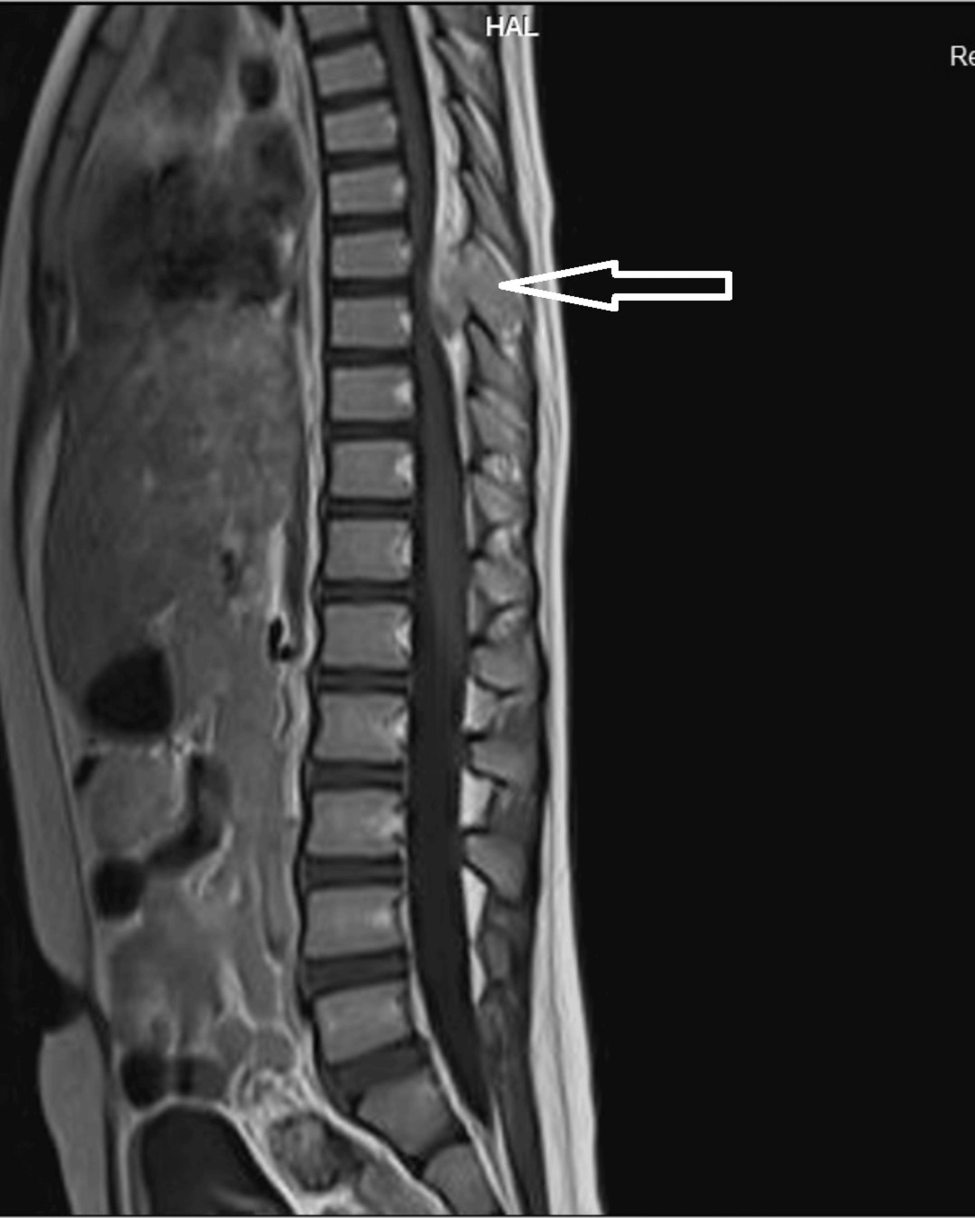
MRI image showing the aneurysmal bone cyst four years prior to admission
